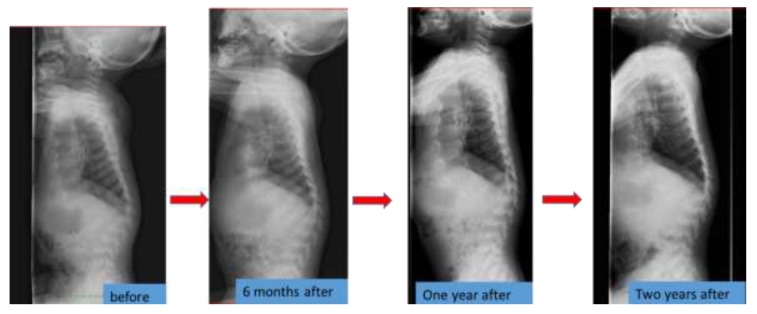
The spine X-ray findings before and after the enzyme replacement therapy in a Japanese boy with MPS IVA.
